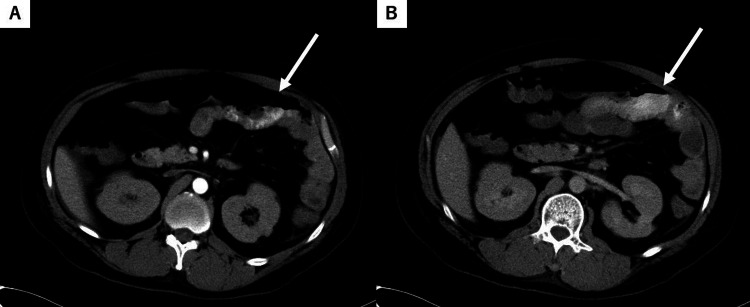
Contrast-enhanced computed tomography on day 23 for case 1. (A) Arterial phase image demonstrates a high-attenuation area in the jejunum, suggestive of active extravasation. (B) Equilibrium phase image shows persistent extravasation with increased distribution of contrast medium, consistent with ongoing small bowel hemorrhage.
